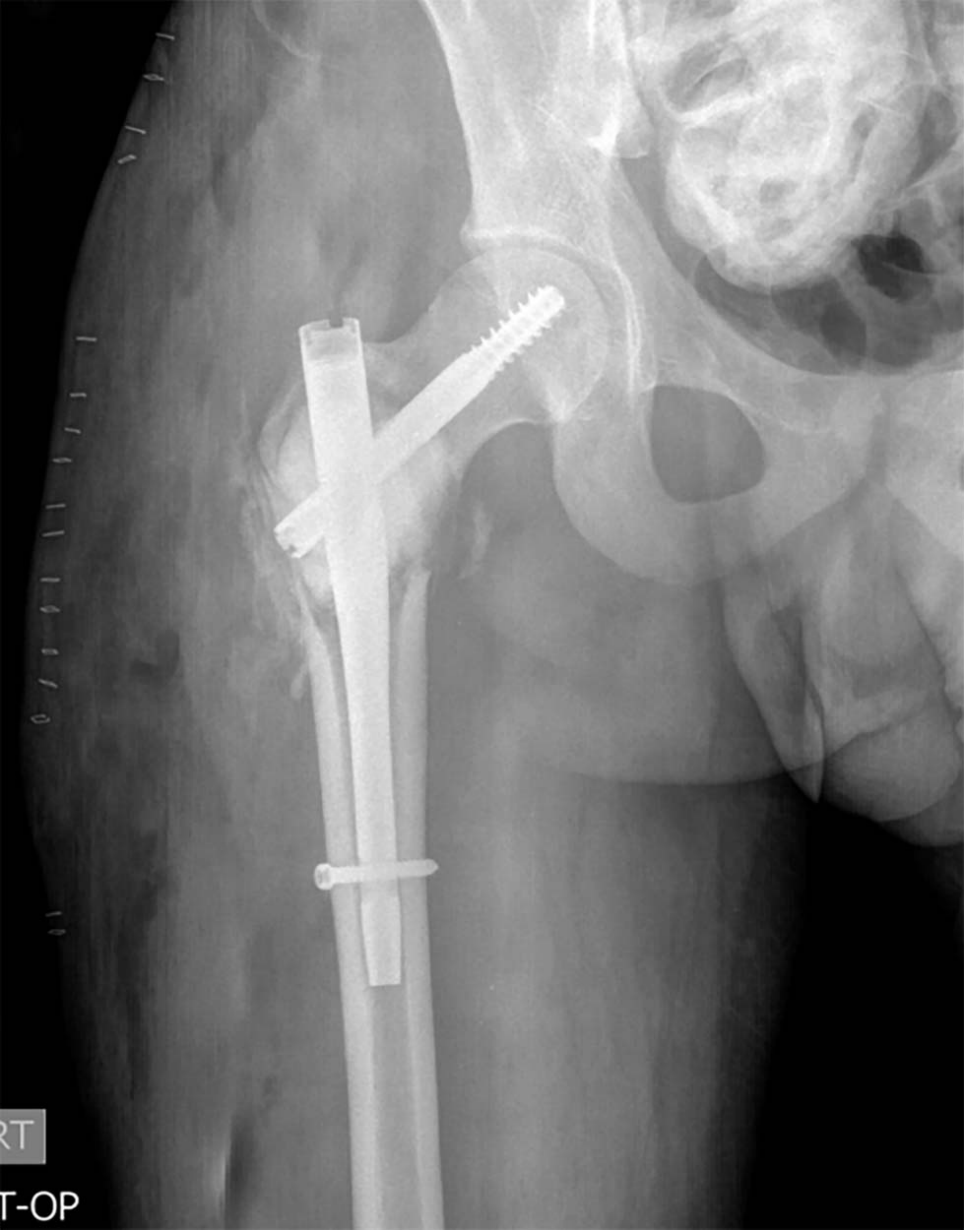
Postoperative AP radiograph of the right hip after intralesional treatment
